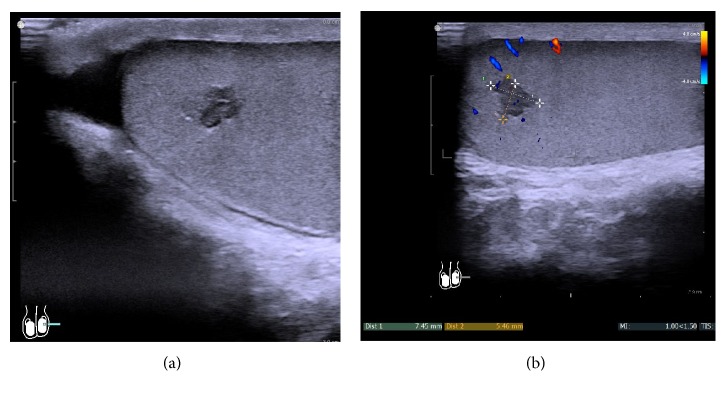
(a) Sonographic appearance of the adult granulosa cell tumor in the 22-year-old patient. Note the lobulated but well circumscribed shape and homogeneous hypoechoic appearance. (b) The same patient, color-coded duplex sonography showing duplex signals within the hypoechoic lesion.
